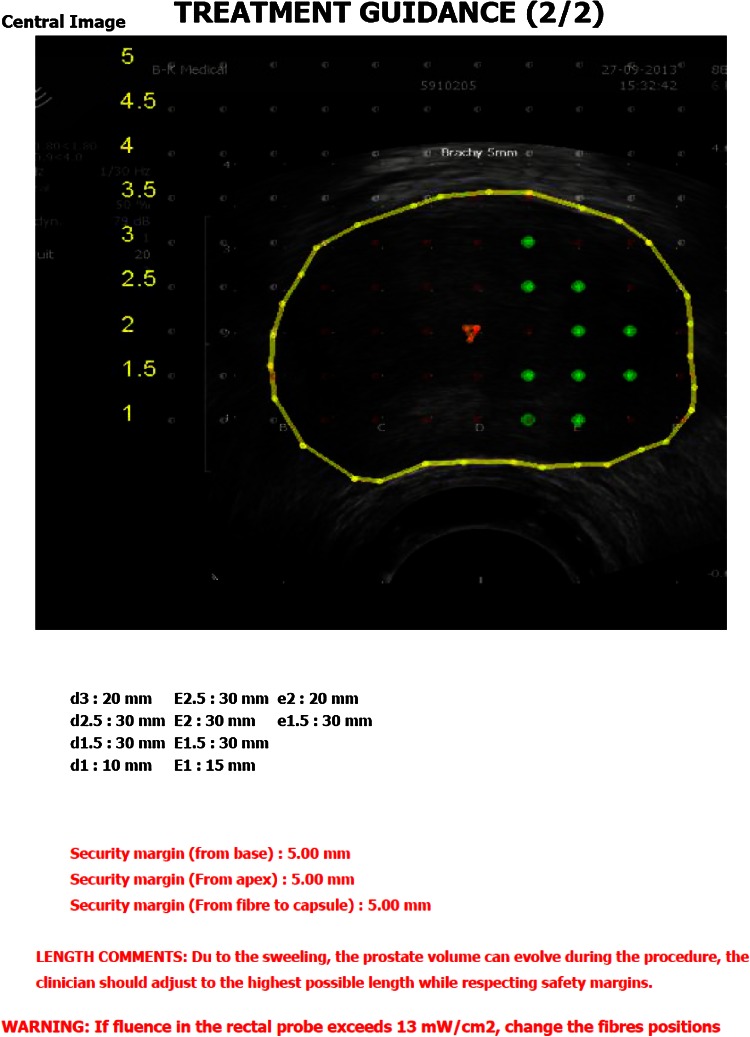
TOOGUIDE^®^ treatment guidance. The position of the fibers is determined on either prostatic MRI/or prostatic ultrasound
